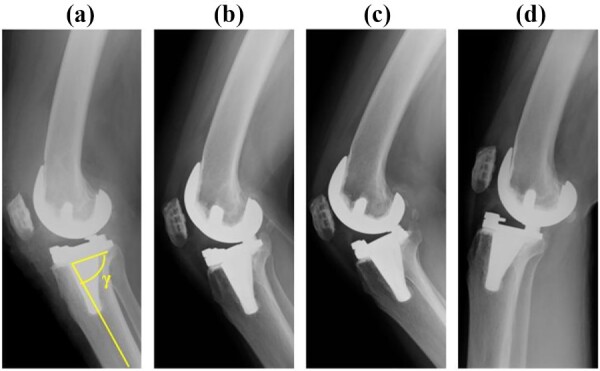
Post-operative anteroposterior and lateral plain radiographs. The γ angle was 82°. (a) Immediate after surgery, (b) 5 years after surgery, (c) 8 years after surgery, (d) 10 years after surgery.
